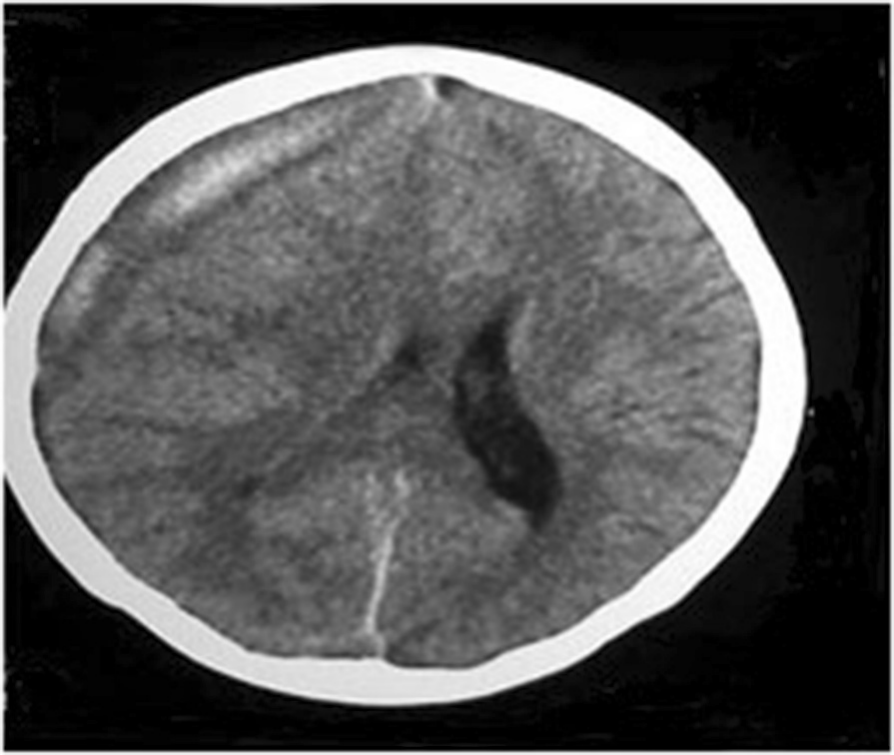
Image of computed tomography scan of brain on postpartum day 36: a right-sided acute on chronic subdural haemorrhage with midline shift and evidence of cerebral oedema.
